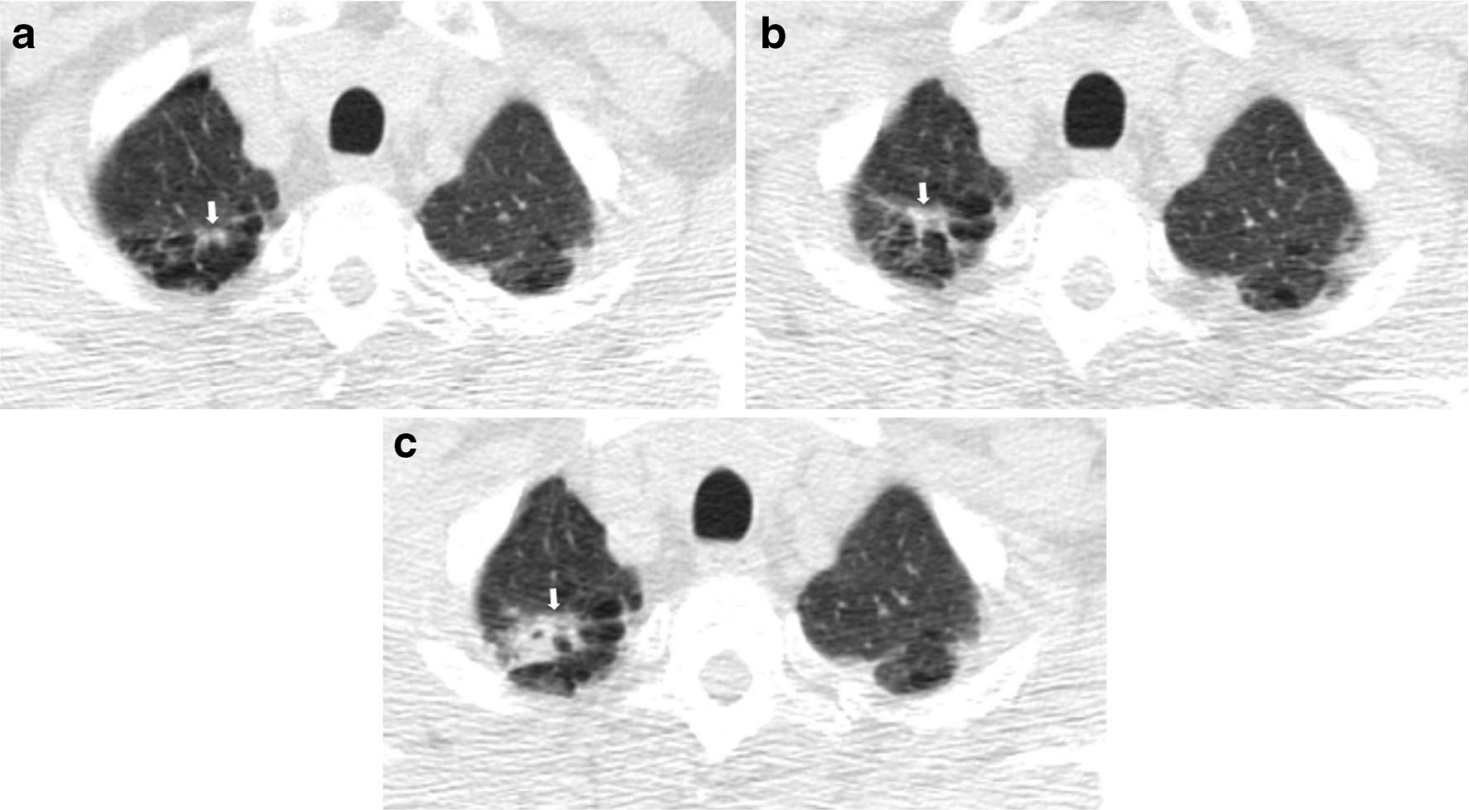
Right apical lung cancer in a 71-year-old man. (a) Low-dose axial CT scan shows small irregular fibrotic changes of the right apex (*arrow*). (b) One year later, LDCT shows a slight increment of the focal abnormalities with the typical aspect of apical fibrotic changes, with a pleural tag and concomitant emphysema (*arrow*). (c) Two years later, a remarkable increment in size was clearly depicted (*arrow*) and the lesion has been surgically removed with a diagnosis of lung adenocarcinoma
